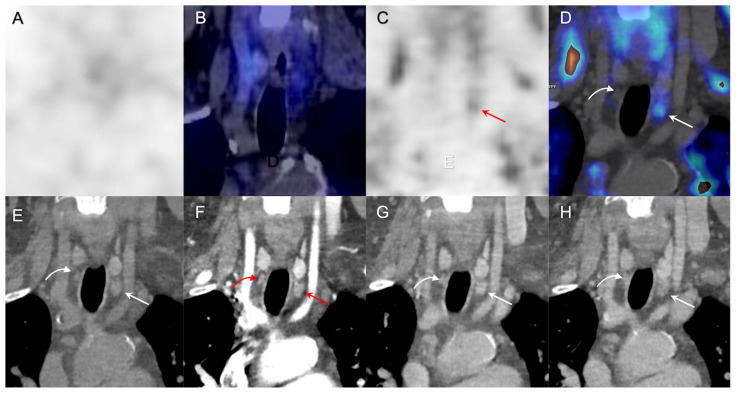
18F-FCH PET/CT and 4D-CT results in a 60-year-old woman with a history of kidney transplantation and dialysis referred for a secondary hyperparathyroidism (PTH: 775 ng/L, calcemia: 2.45 mmol/L). From left to right: coronal SPECT (A), coronal SPECT/CT (B), coronal PET (C), coronal PET/CT (D), non-contrast-enhanced CT (E), arterial-phase CT (30 s after injection) (F), venous-phase CT (45 s after injection) (G), and late-venous phases (70 s after injection) (H). Preoperative parathyroid scintigraphy and US failed to detect hyperfunctioning gland, and 18F-FCholine PET/CT showed increased focal uptake only in the left inferior parathyroid (arrow). 4D-CT revealed an additional right inferior pathological parathyroid (curbed arrow). The patient underwent bilateral neck exploration, and both left and right inferior parathyroidectomy. Pathology showed parathyroid hyperplasia in both glands. Reduction of preoperative PTH-serum concentration was greater than 80%. The patient showed a normal biological profile during her 12 months post-surgical follow-up.
